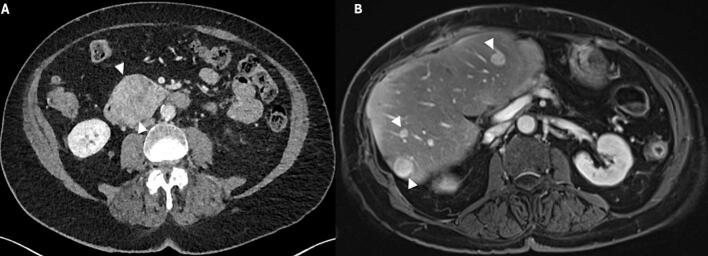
(A) Portal venous phase abdominal CT in axial direction, showing a mass (between arrowheads) in the pancreatic head with a maximum diameter of 57 mm and heterogeneous enhancement. (B) Contrast-enhanced abdominal MRI in axial direction in the portal venous phase, showing several enhancing nodular lesions (arrowheads) diffusely distributed in the liver, suspicious for liver metastases.
